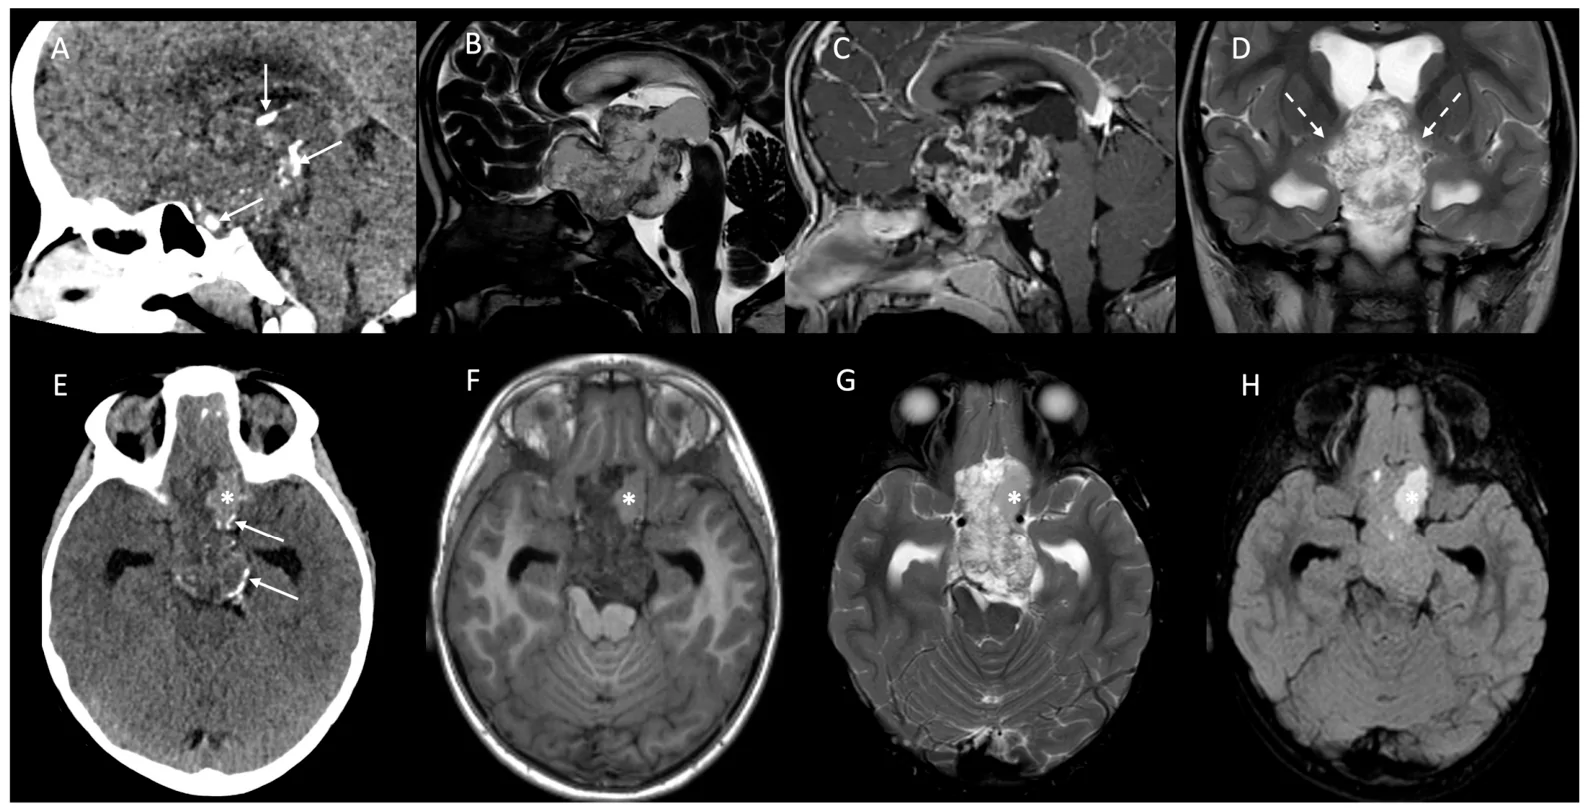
ACP. CT and MR studies of a 7-year-old boy with headache and vomiting. (A) Sagittal CT, (B) sagittal bSSFP, (C) sagittal contrast-enhanced 3D T1, (D) coronal T2, (E) axial CT, (F) axial 3D T1, (G) T2, and (H) FLAIR. Multiloculated sellar/suprasellar solid/cystic lesions involving the third ventricle with obstructive hydrocephalus and compression of the hypothalamus (D, dashed arrows). The lesion is characterized by inhomogeneous cystic content and strong enhancement of solid components and cystic walls. Note multiple intratumoral and peripheral calcifications (A,E, arrows) and the presence of hyperdense and T1/T2/FLAIR hyperintense proteinaceous cystic fluid (E–H, asterisks).
